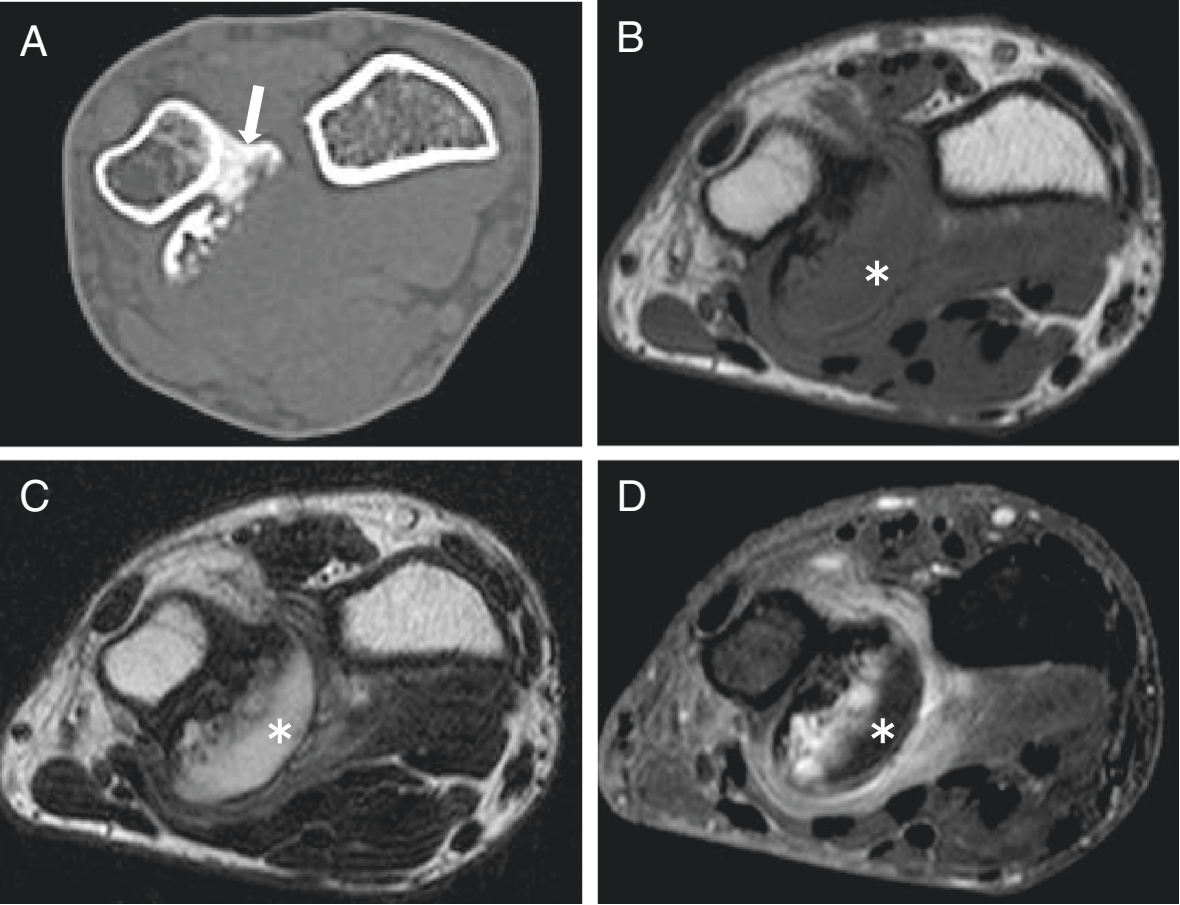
CT and MRI findings. a The CT scan shows a pedunculated bony prominence arising from the distal end of the ulna that has no continuity with the medullary cavity (arrow). b A axial T1-weighted MRI image that shows a low signal intensity at the margins of the lesion (asterisk). c A T2-weighted image that shows a high signal intensity at the margins of the lesion (asterisk). d A gadolinium-enhanced image shows a low signal intensity at the margins of the lesion (asterisk)
